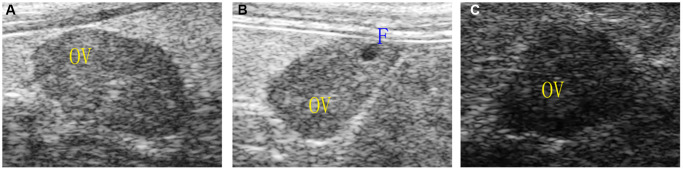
Ovarian morphology under ultrasound. (A) Normal group; (B) Natural aging non-intervention group; (C) Natural aging intervention group; OV, ovary; F, follicle.
